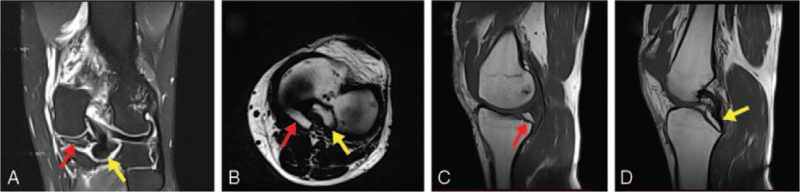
Preoperative magnetic resonance images findings of the knee (A) Coronal T2 FS image, the red arrow shows avulsion fracture of the medial meniscus, and yellow arrow shows avulsion fracture of the posterior cruciate ligament (PCL). (B) Axial T1 image, the red arrow shows avulsion fracture of the medial meniscus, and yellow arrow shows avulsion fracture of the PCL. (C) Sagittal PD image, the red arrow shows avulsion fracture of the medial meniscus. (D) Sagittal PD image yellow arrow shows avulsion fracture of the PCL.
